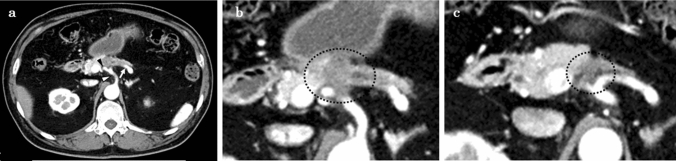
Enhanced computed tomography findings of the primary tumor. a: Enhanced CT showed the tumor involving the CHA (black arrowhead), SA (white arrow), and CA (white arrowhead) at the first visit. b: Magnified enhanced CT at the first visit showed that the tumor (black dotted circle), 24 mm in diameter. c: After four cycles of chemotherapy, enhanced CT showed that the tumor size had decreased 16 mm in diameter, and the soft-density mass (black dotted circle) around the CHA, SA, and CA had shrunk. *CA* celiac artery, *CHA* common hepatic artery, *CT* computed tomography, *SA* splenic artery
